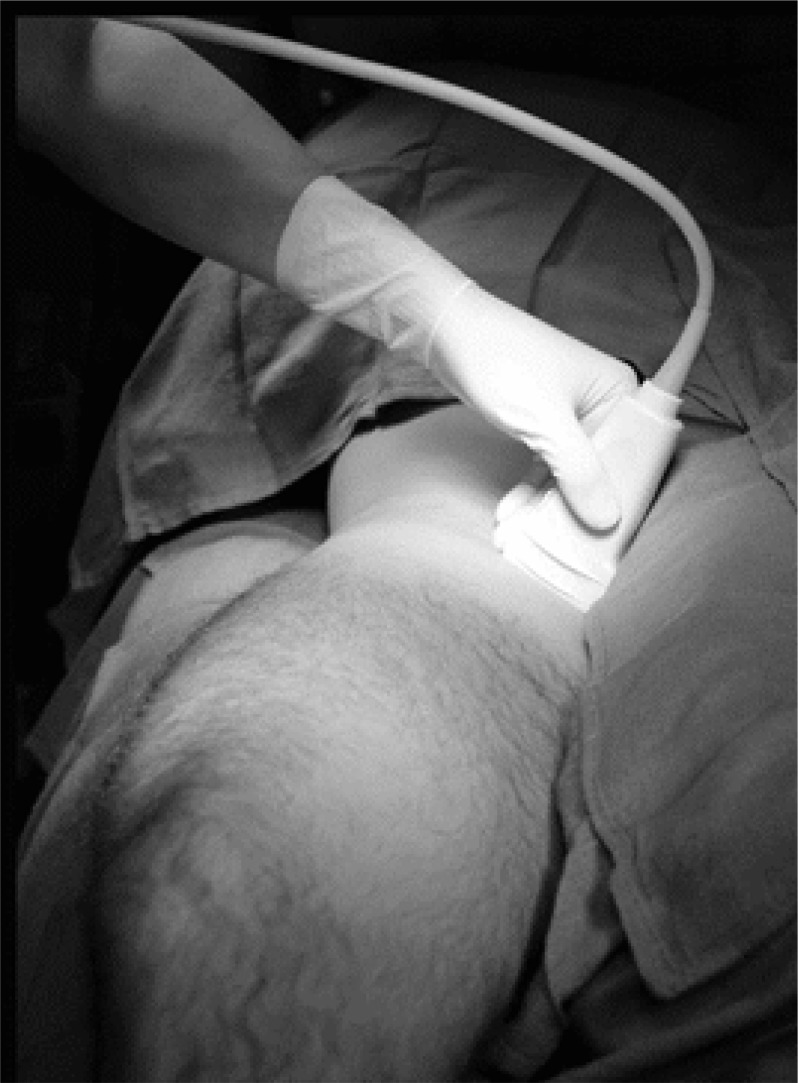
Position of ultrasonography probe in inguinal area
